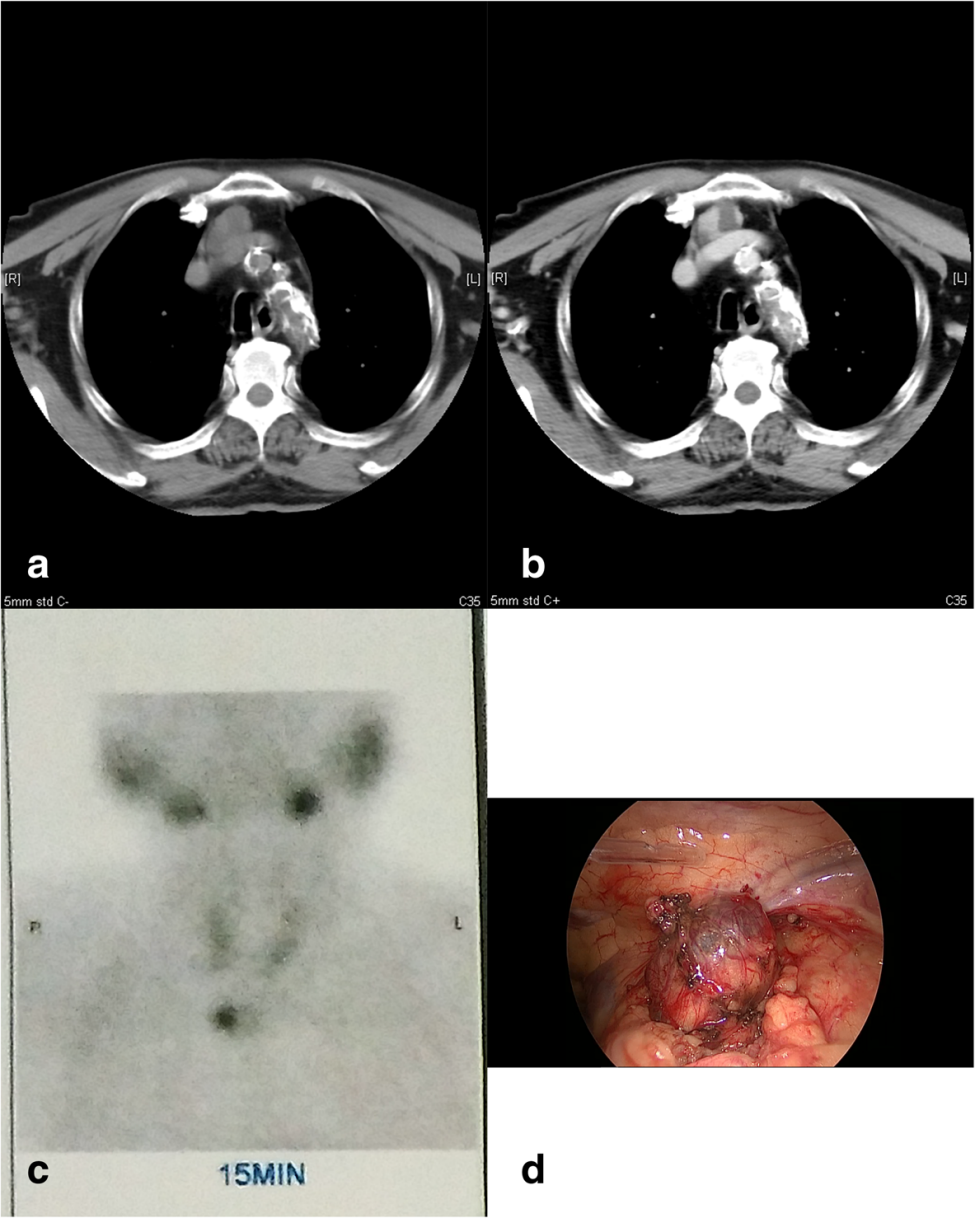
a A neck CT scan had revealed a 2.5*1.9 cm mass(arrow) in the upper region of anterior mediastinum; b A contrast-enhanced neck CT scan had displayed nodular mixed density; c Tc-99 m dual-phases parathyroid scan had detected a hyperfunctioning parathyroid gland(arrow) in the upper region of anterior mediastinum; d A video-assisted thoracoscopy had showed a smooth and nonulcerated nodular lesion(arrow) immediately below the normal mucosa
